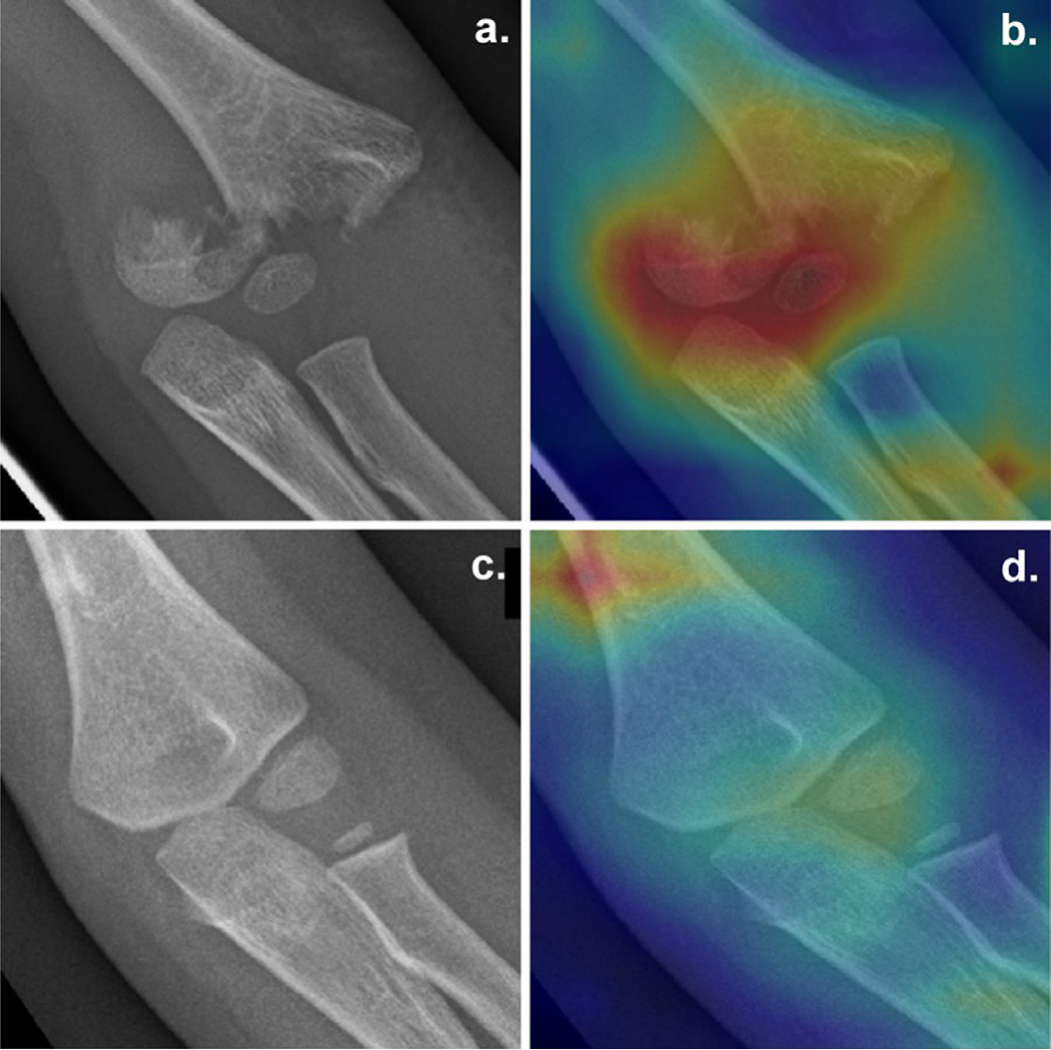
a,b: Example of true-positive Grad-CAM with pallet humeral frontal X-ray in a 4 year old boy and corresponding Grad-CAM using M1; c,d: Example of false-positive case: supracondylar process which was inadequately considered as a fracture by the model in a 6-year old girl using M1.
